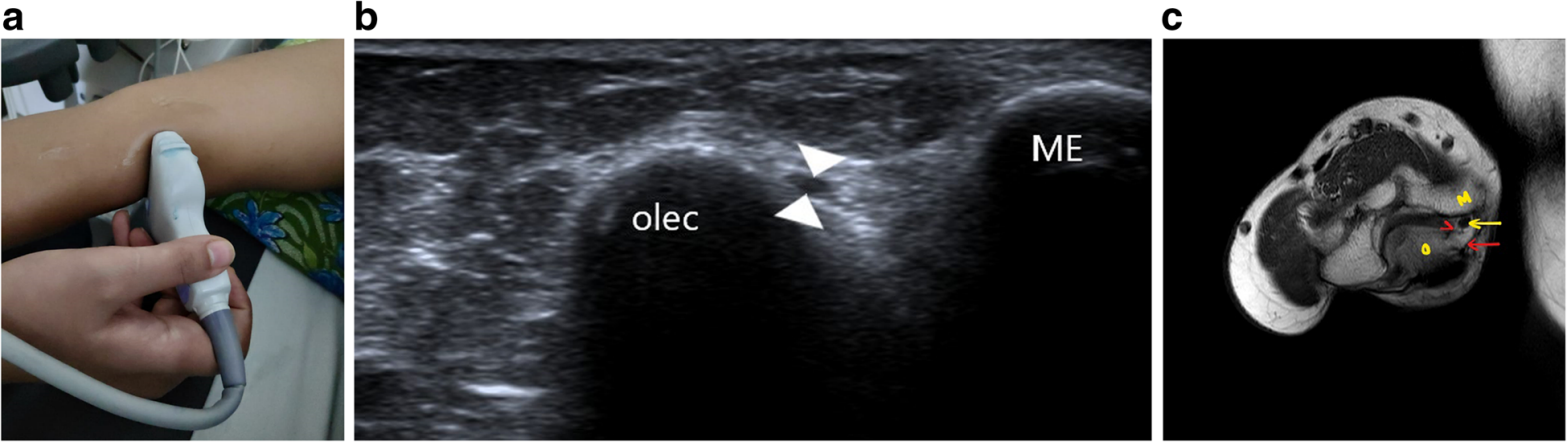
a Probe position for localisation of the ulnar nerve at the elbow. b The nerve (arrowhead) is localised in its short axis within the cubital tunnel between the medial epicondyle (ME) and olecranon process (olec). c MR image showing the normal ulnar nerve (yellow arrow) in the cubital tunnel: medial epicondyle (M) and olecranon process (O) forming the medial and lateral walls, Osborne’s ligament (red arrow) forming the roof and the floor being formed by the joint capsule of elbow and the medial collateral ligament (red arrowhead)
